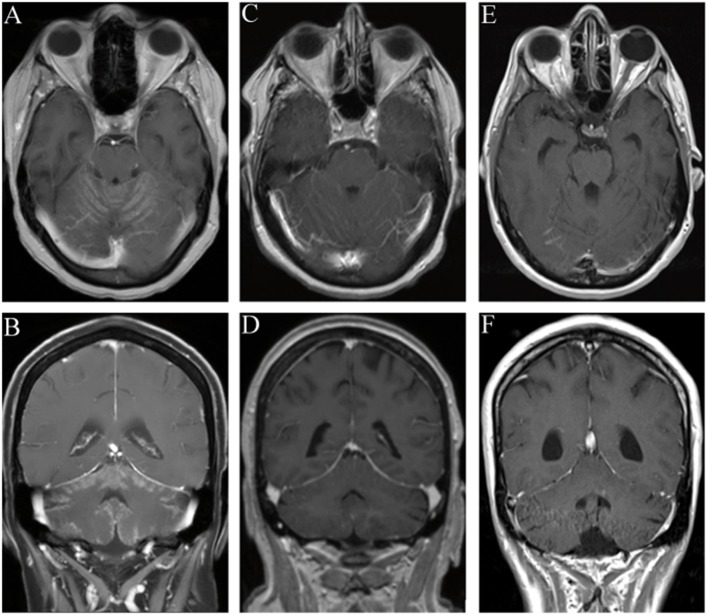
Representative MRI findings of patients with leptomeningeal metastasis. In all patients malignant cells were found in the CSF. (A,B) Gadolinium-enhanced coronal and axial T1-weighted images demonstrate leptomeningeal enhancement. CSF diagnostic revealed pleocytosis with 12 cells/μl. (C,D) Leptomeningeal enhancement in Gadolinium-enhanced coronal and axial T1-weighted images in a patient with normal CSF cell count. (E,F) No leptomeningeal enhancement could be detected in Gadolinium-enhanced coronal and axial T1-weighted images in a patient with CSF pleocytosis (7 cells/μl).
